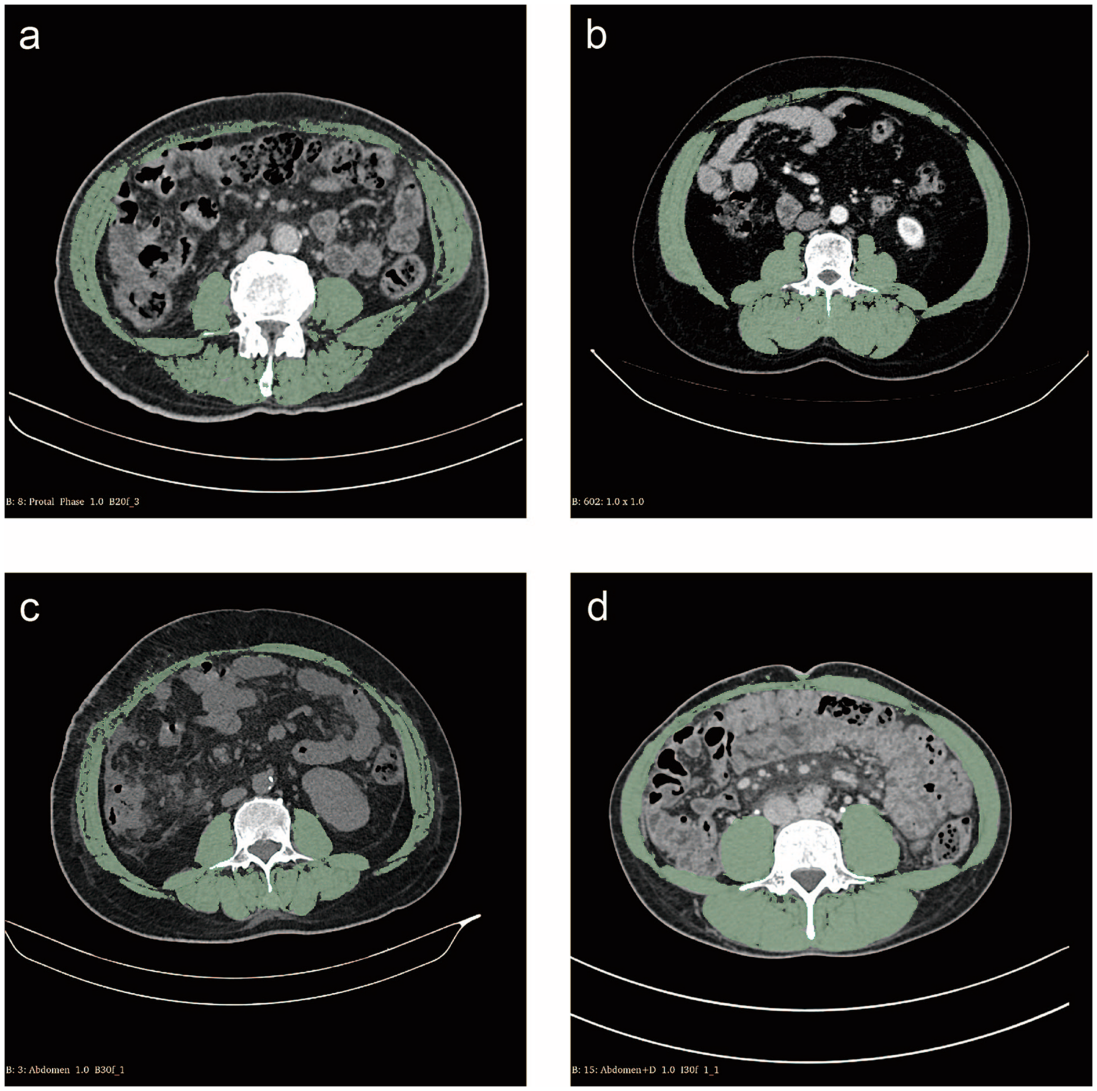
Cross-sectional computed tomography measurement of skeletal muscle areas (green) at the L3 vertebral layer. (a) Patient with sarcopenia of skeletal muscle index (SMI) 38.74 cm^2^/m^2^; (b) Patient without sarcopenia of SMI 61.99 cm^2^/m^2^; (c) Patient with myosteatosis of skeletal muscle density (SMD) 35.53 HU; (d) Patient without myosteatosis of SMD 56.62 HU.
